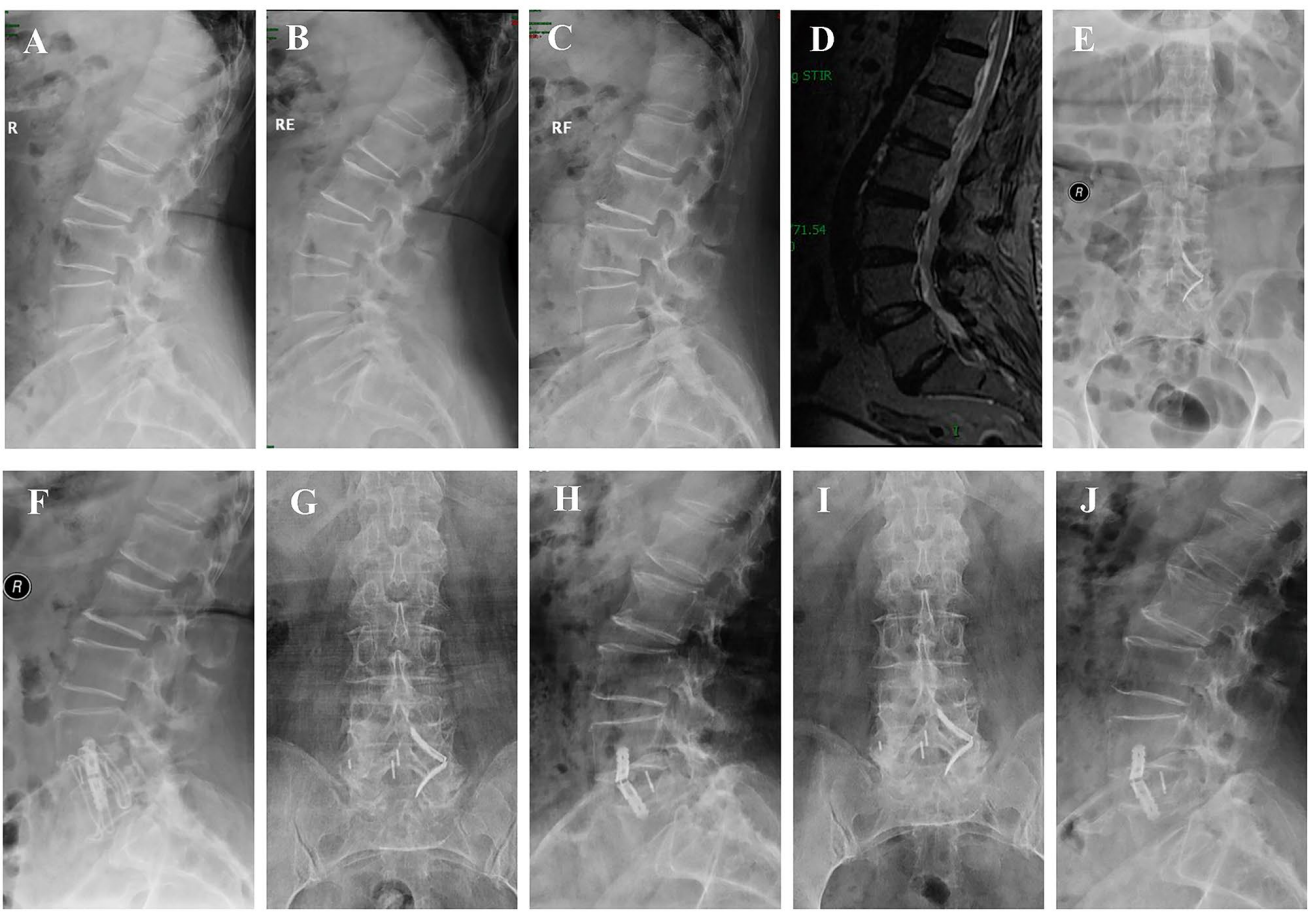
Pre-, Post-operative and follow-up images obtained in a 71-year-old female who had low back pain with radiating pain in the left lower extremity, exacerbated during exercise and presented with L4-5 degenerative spondylolisthesis. The symptoms were significantly improved after SA-LLIF. (A) The lateral X-ray showed lumbar degenerated spondylolisthesis; (B, C) The flexion-extension lateral X-rays showed L4-5 spondylolisthesis which exacerbated in the extension position; (D) MRI fat-suppressed image showed L4-5 intervertebral disc degenerated spondylolisthesis; (E, F) The postoperative AP and lateral X-rays shows recovery of L4-5 vertebral alignment; (G, H) 3 months after operation, the lateral X-ray shows that the upper endplate of the distal vertebral body collapses 2 mm, and the cage subsided. But the position of the plate and cage were stable; (I, J) 12 months after operation, the lateral X-ray shows the plate and cage were still stable
